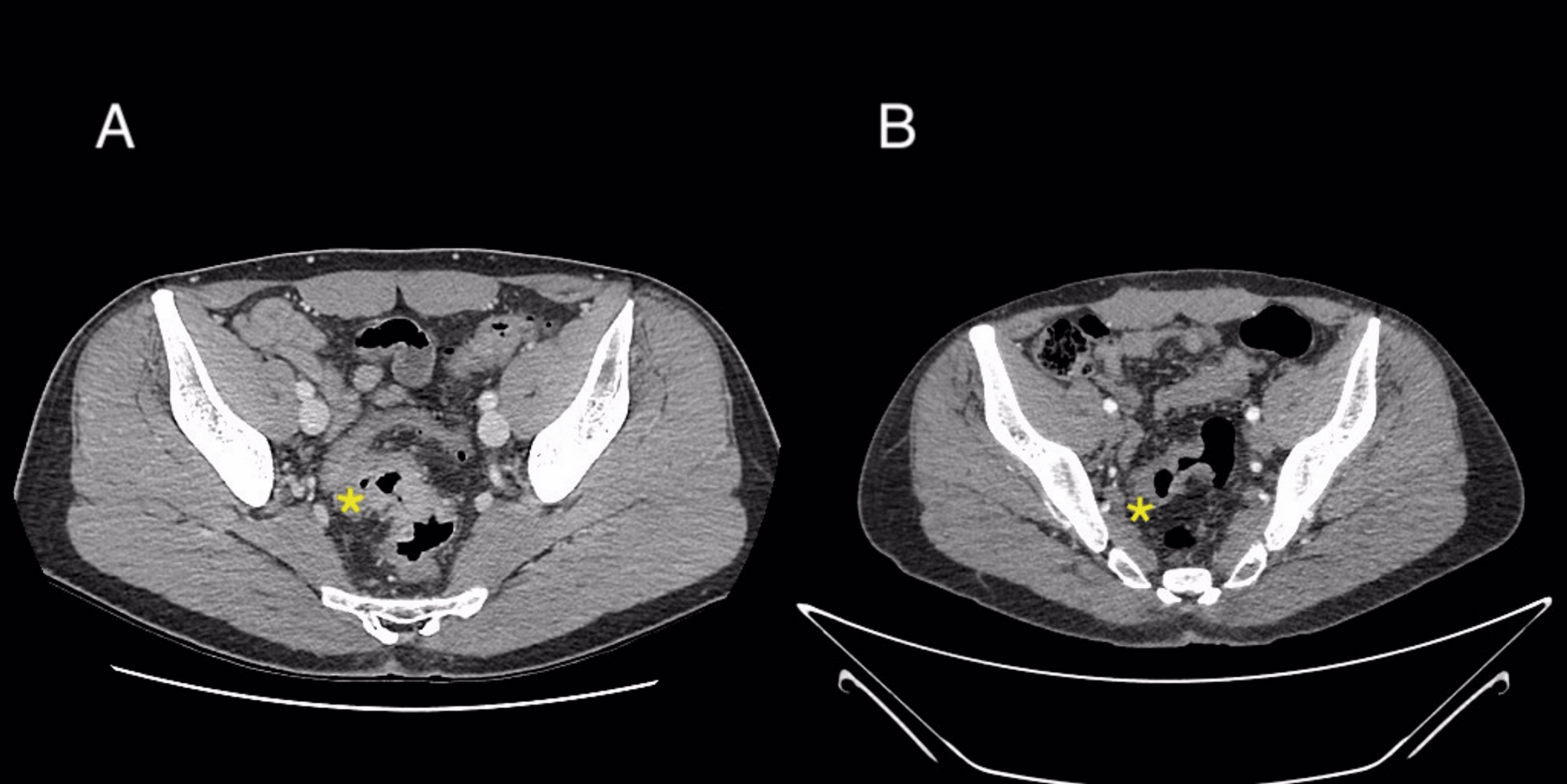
CT scan axial plane A: Prior to chemotherapy treatment. B: Following chemotherapy treatment. CT scan showed a significant reduction in the thickness of the parietal thickening of the rectosigmoid, now measuring 6 mm compared to 9 mm. CT: computed tomography
